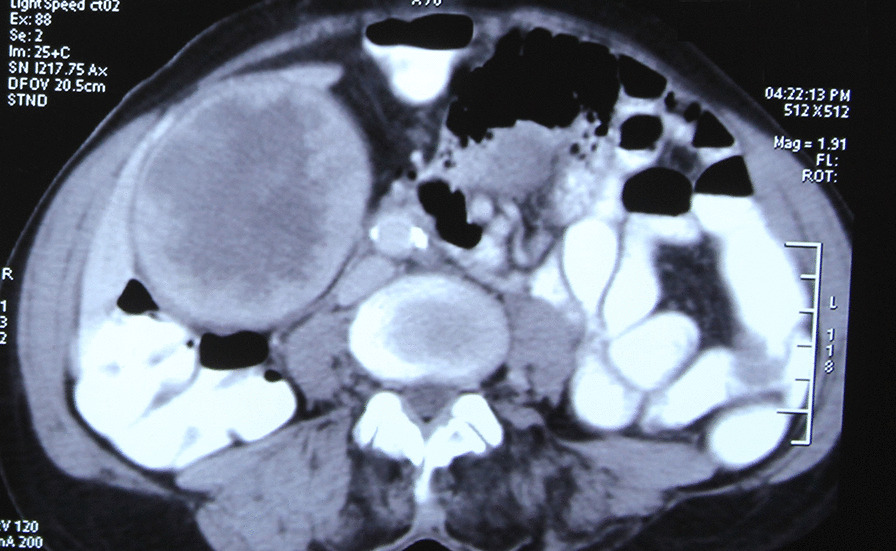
CT scan (transverse plane) showing thickened irregular GB wall
